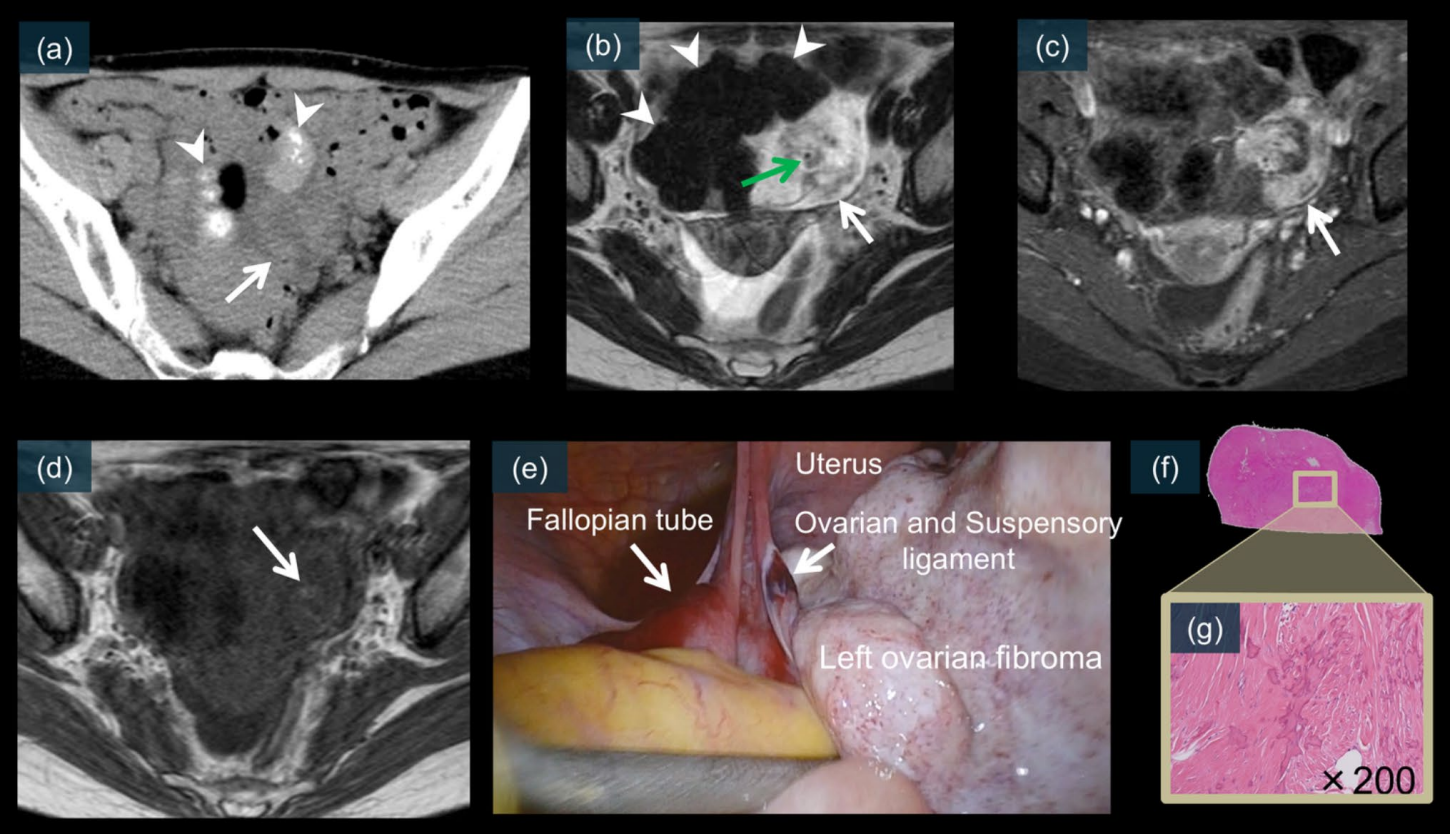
A 48-year-old female. a is an unenhanced CT image showing the superior portion of the ovarian fibroma with strong calcification (white arrowheads), adjacent to which a mass-like swelling is observed (white arrow). On T2WI (b), a thickened, edematous pedicle (white arrow) is observed adjacent to a low-signal mass suggestive of left ovarian fibroma (white arrowheads). A flow void suspected to be the ovarian artery is visible in the center of the pedicle (green arrow). This area shows a relatively strong enhancement (c, white arrow). On T1WI (d), a partially high-signal area was observed, suggesting hemorrhage (white arrow). e is a laparoscopic image revealing a 720° torsion of the left ovarian fibroma and fallopian tube. Pathological examination showed abundant thick collagen fibers with calcification deposits (f and g)
